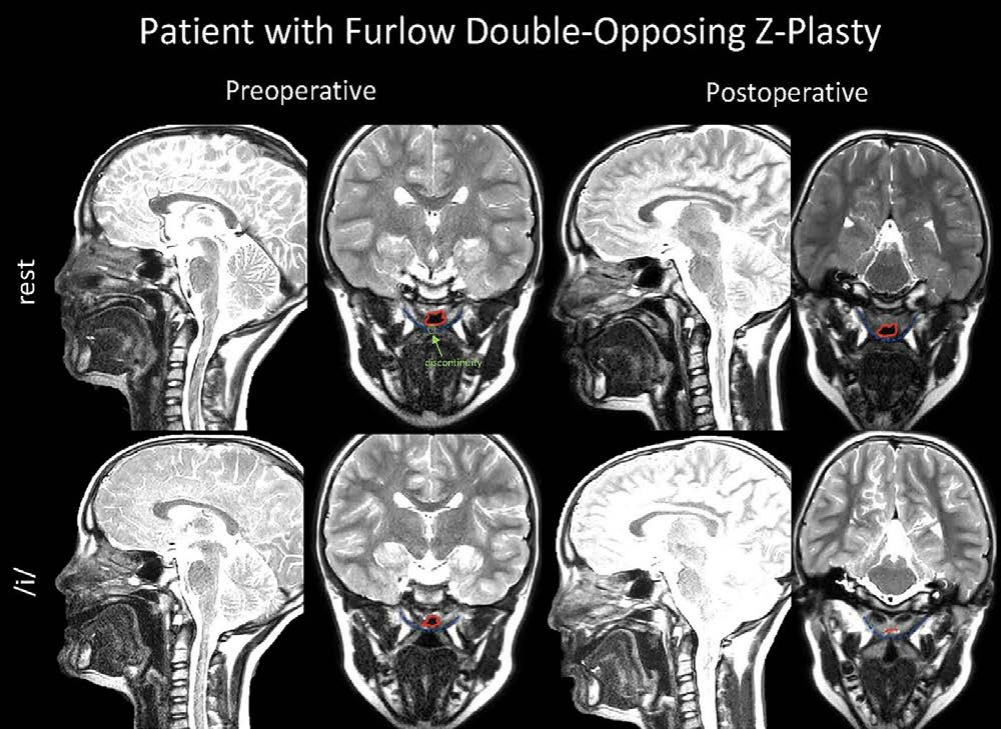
Preoperative (left) and postoperative (right) MRI images of a patient who underwent Furlow double-opposing Z-plasty. Images are taken at rest (top) and during sustained phonation of/i/ (bottom) in the sagittal and oblique coronal views. The velopharyngeal port is outlined in red. The levator veli palatini is outlined in blue. Levator discontinuity is shown in green.
